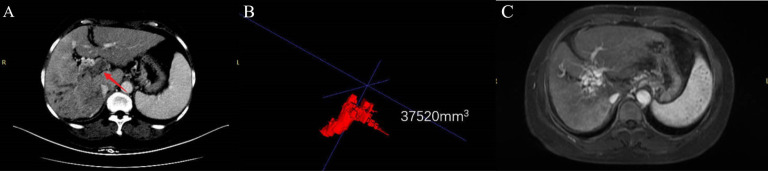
A typical case. A 55-year-old female patient was diagnosed with advanced hepatocellular carcinoma with VP4. Combined with the pre-treatment panel (A), contrast-enhanced CT in the portal venous phase was obtained, showing both the main portal vein and its right branch Filled with PVTT (arrow). Panel (B) is the PVTT image delineated by ITKs, and the PVTT volume is obtained to be 37520 mm3. Panel (C) is an enhanced MR image after 5 cycles of HAIC (mFOLFOX) combined with donafenib and camrelizumab. It shows that the portal vein tumor thrombus disappeared and portal vein cavernous changes appeared, and the PVTT response reached CR.
